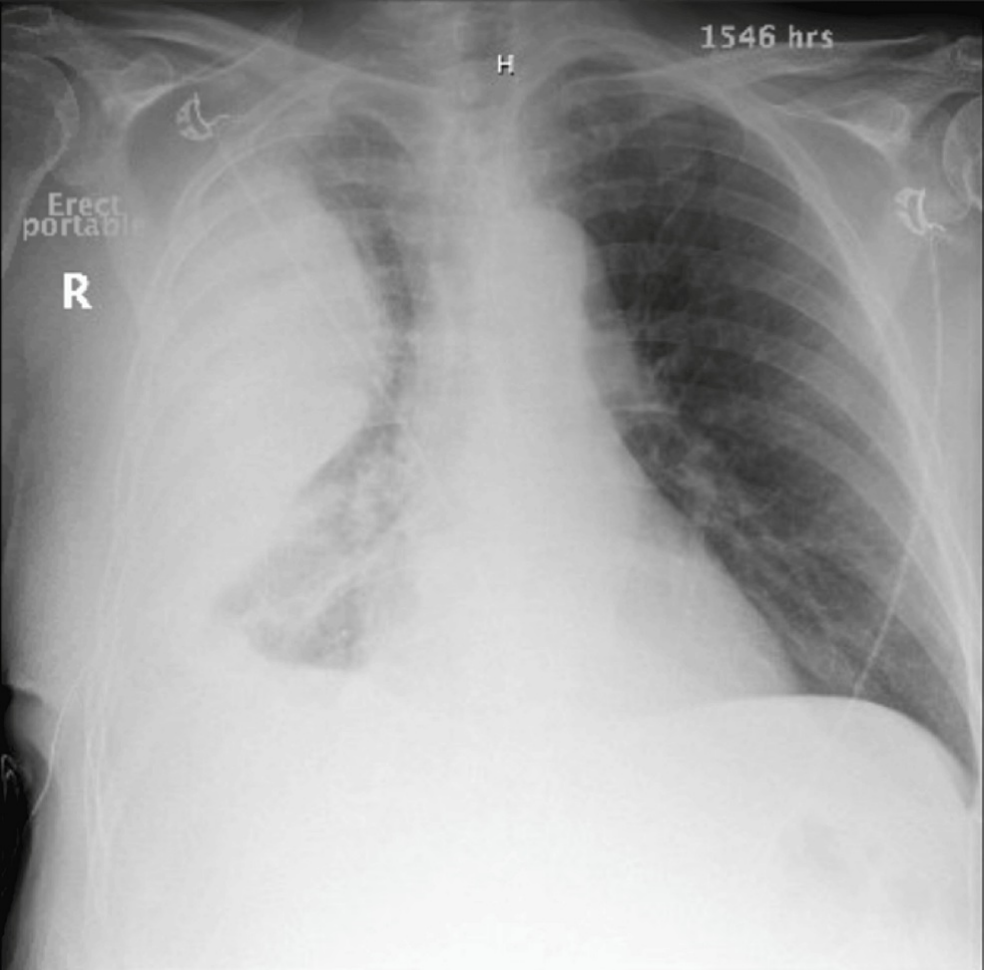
AP CXR shows a large loculated right pleural effusion with a drastic interval increase AP: anteroposterior; CXR: chest X-ray
